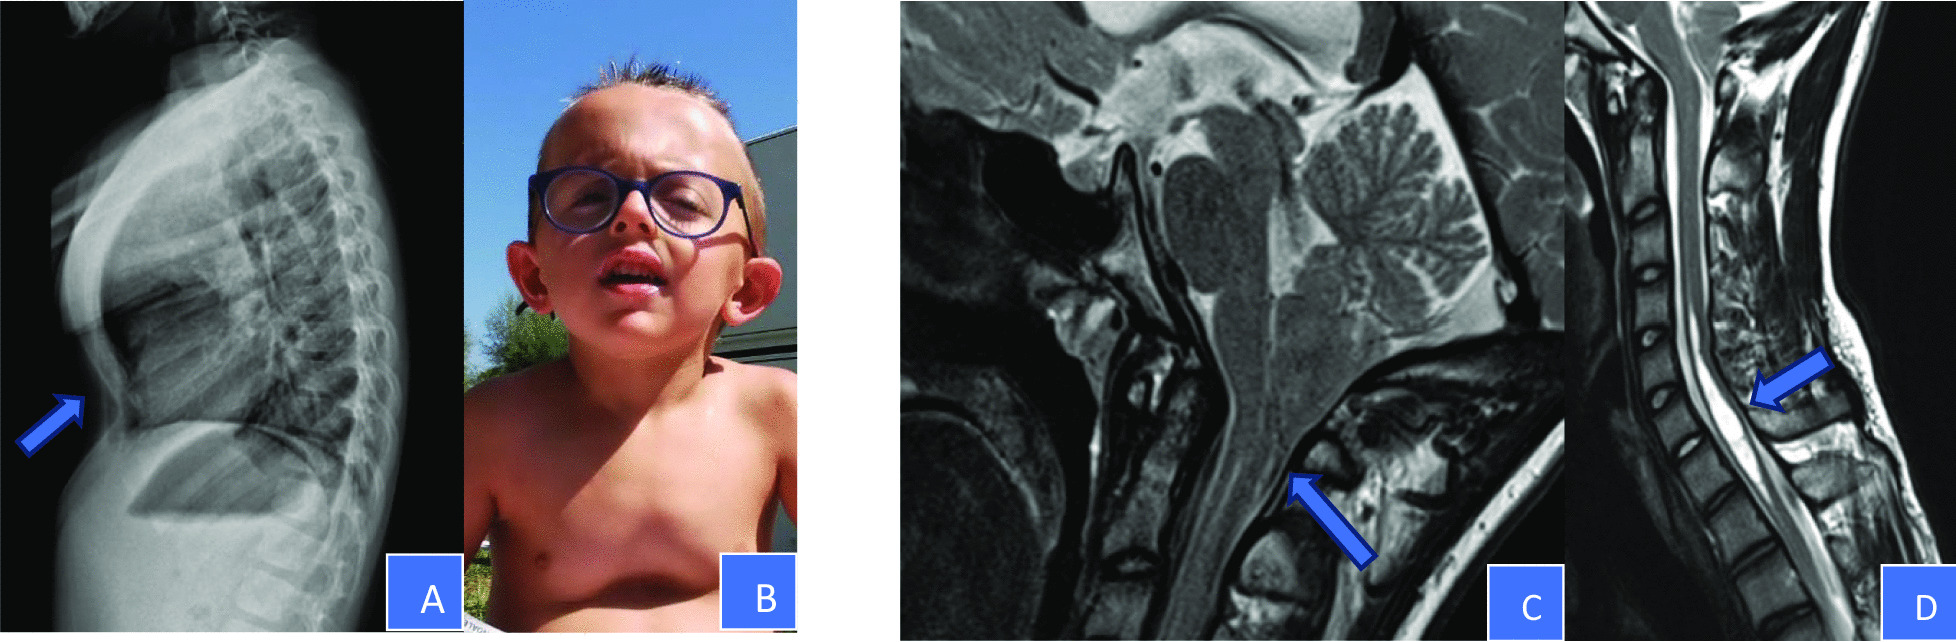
Musculoskeletal anomalies: A and B skeletal Xray and photo of case 16, that showed marked kyphosis with pectus carinatum in the upper half of the sternum and excavatum in the lower half. C and D MRI performed on case 1. The patient presented a Chiari malformation type I with a protrusion of cerebellar tonsils through the foramen magnum of 11 mm. Presence of syringomyelic cavity in the cervical spine, affecting the tract C5–C7
